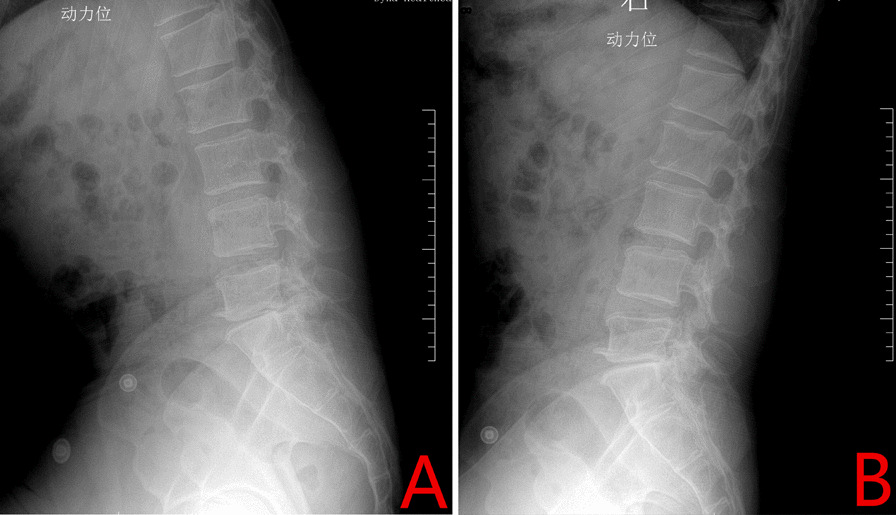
Preoperative radiography. A Preoperative flexion X-ray image. B Preoperative hyperextension X-ray image. Lumbar spine flexion can be seen to be much reduced prior to the operation with decreased gap between the 5th lumbar and the 1st sacral vertebral bodies
